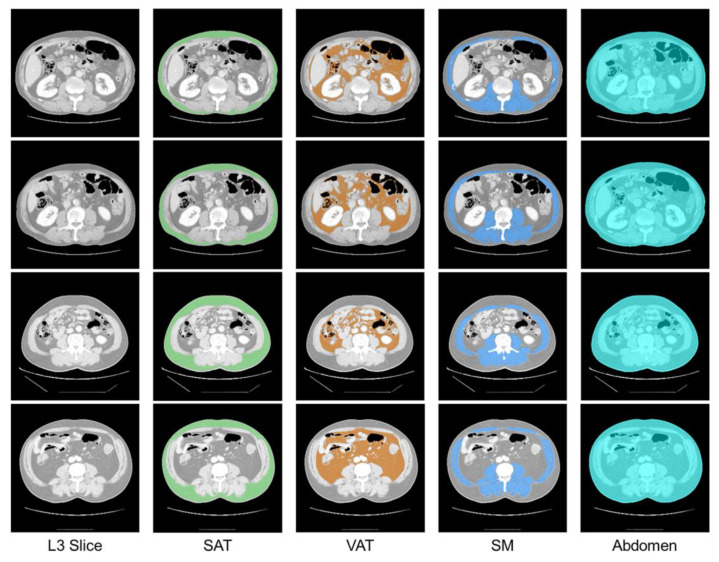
Samples of CT scans with manually segmented abdominal tissues. From left to right, each column presents the original L3 slice, segmented subcutaneous adipose tissue (SAT, light green), visceral adipose tissue (VAT, orange), skeletal muscle (SM, blue), and the combined abdominal segmentation (cyan). Each tissue type is color-coded to enhance visual distinction.
